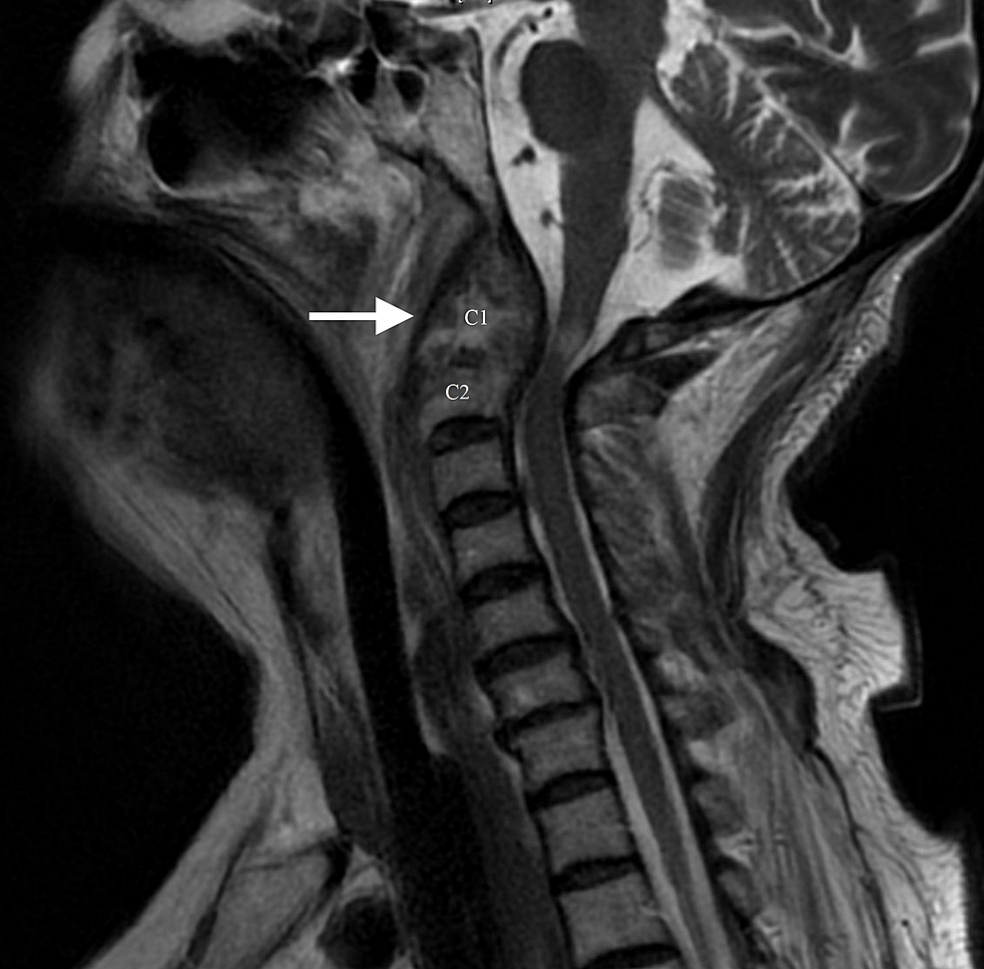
MRI cervical spine reveals pannus formation (arrow) at C1-C2 region. MRI: magnetic resonance imaging C1: cervical vertebra one C2: cervical vertebra two
